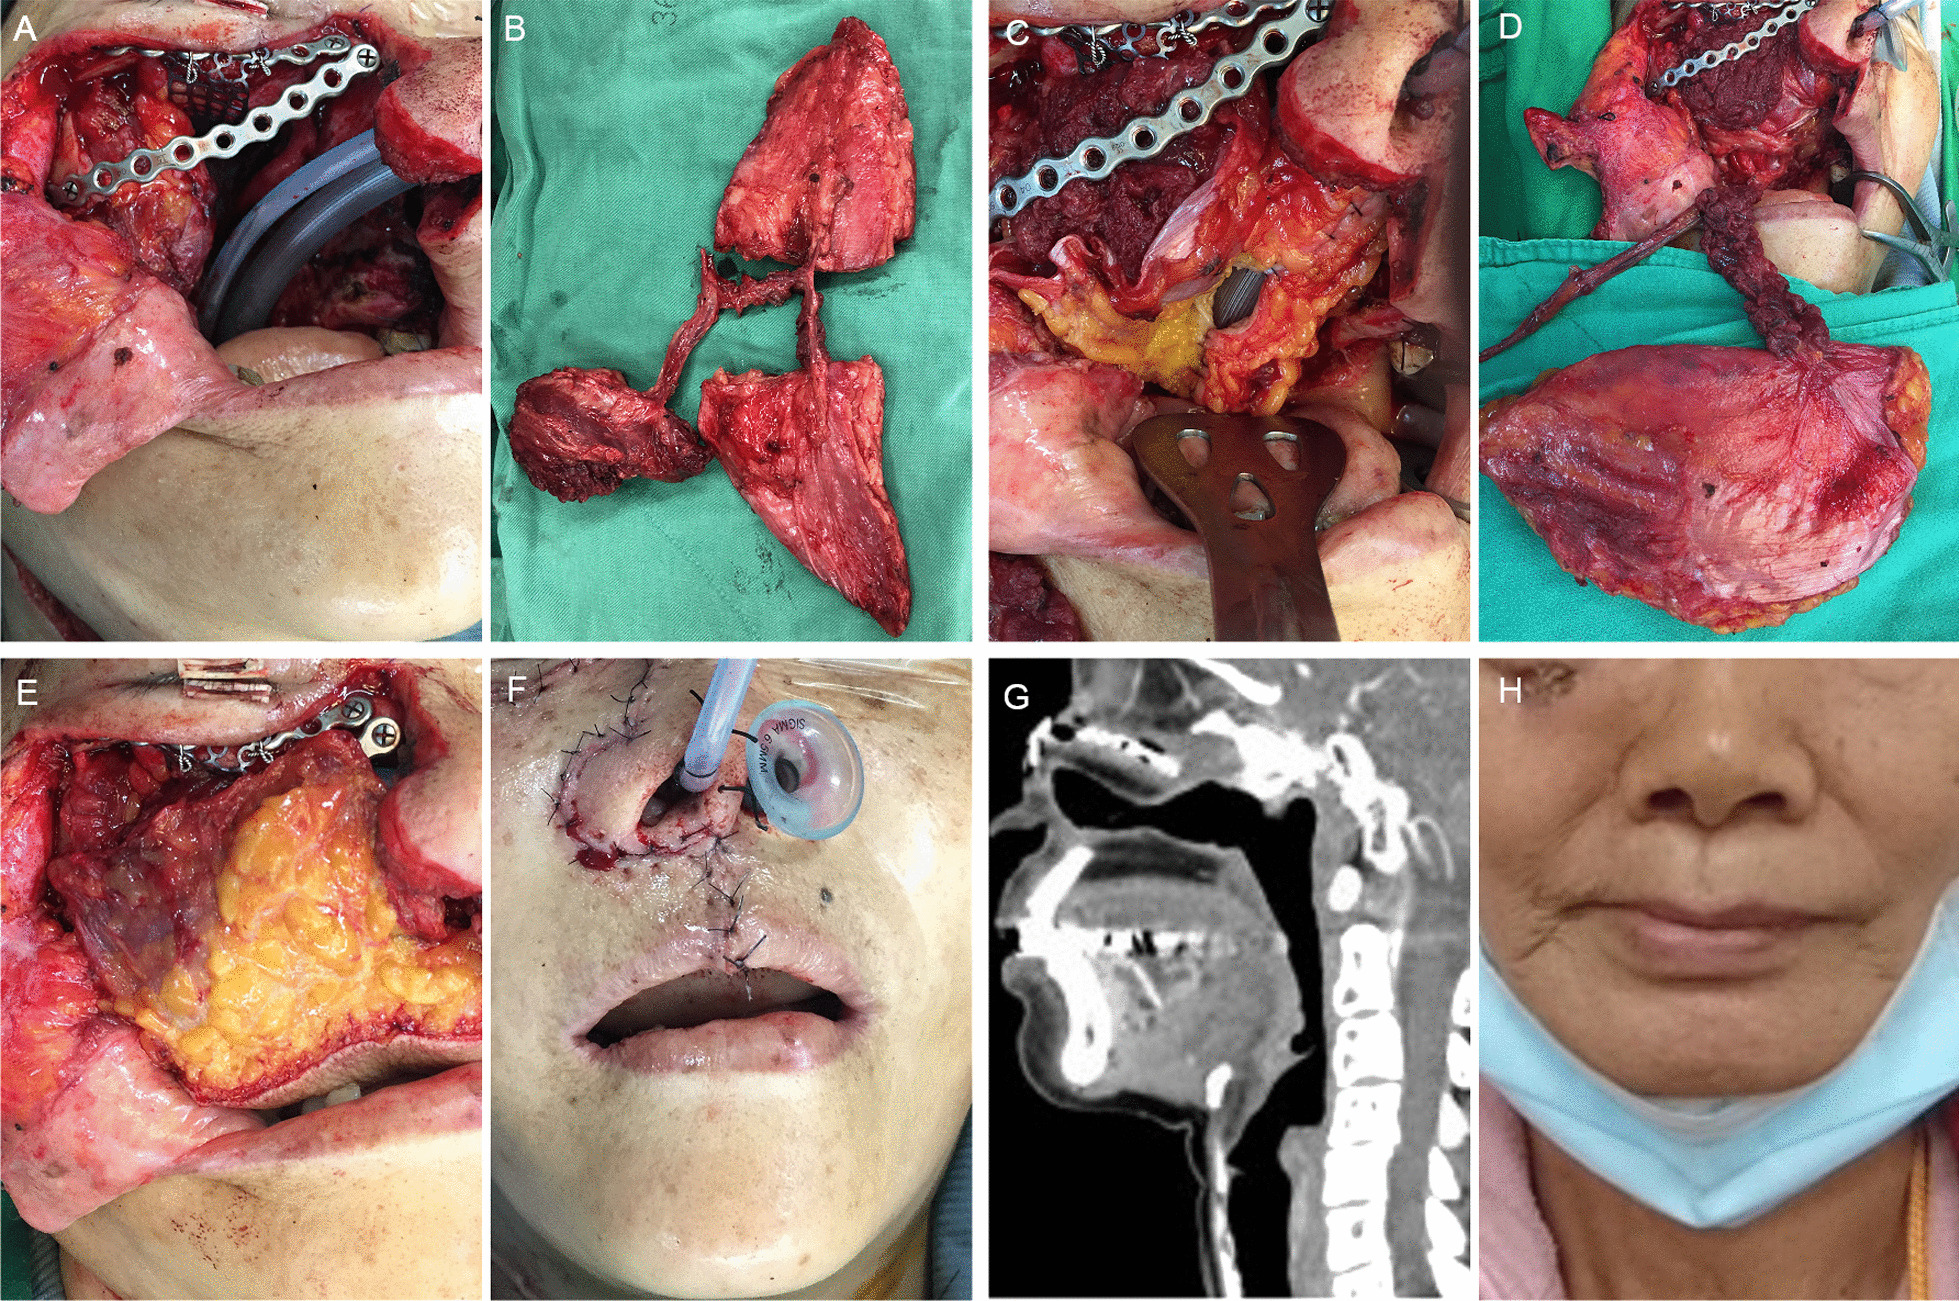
A 45-year-old female patient with maxilla squamous cell carcinoma (T4N0M0) underwent a wide excision and reconstruction of mesh and mini-plates (A). The chimeric ALT flap (30 cm × 6 cm) was designed for simultaneous nasal airway and palatal defect reconstruction (B). A nasal airway tubing surrounded by a skin paddle was used to guide airway reconstruction (C). The VL muscle was transferred to the right maxillary defect (D), and the palatal defect was covered with the other skin paddle in accordance with the defect size (E). An immediately postoperative image is shown (F). At 2-years follow-up, a computed tomography scan revealed partial nasal airway stenosis without compromising the respiratory function (G). Postoperative 2-years follow-up image (H)
